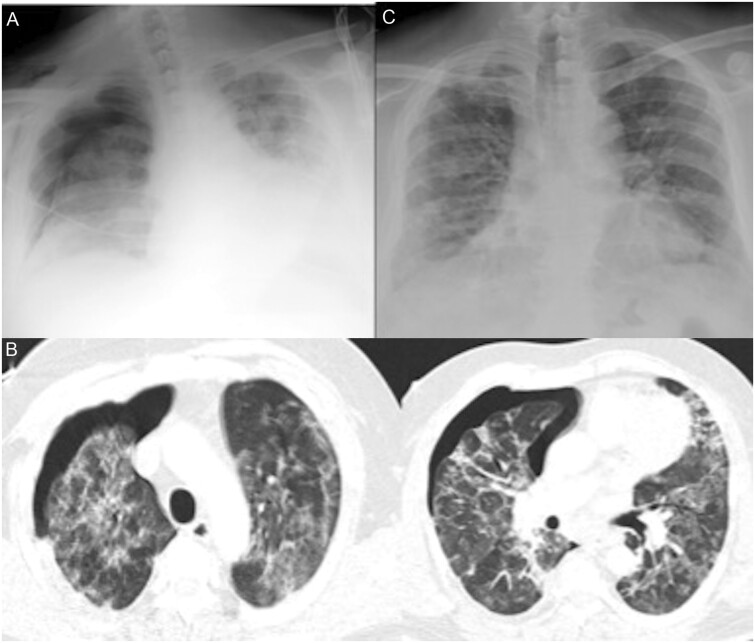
(A) Pneumothorax in the right lung was seen on chest radiography of the patient. (B) Thorax computed tomography of the patient revealed predominantly irregular ground-glass opacities in the peripheral zone and accompanying interstitial thickening in both lungs and pneumothorax on the right. (C) His lungs observed expanded in chest X-ray.
